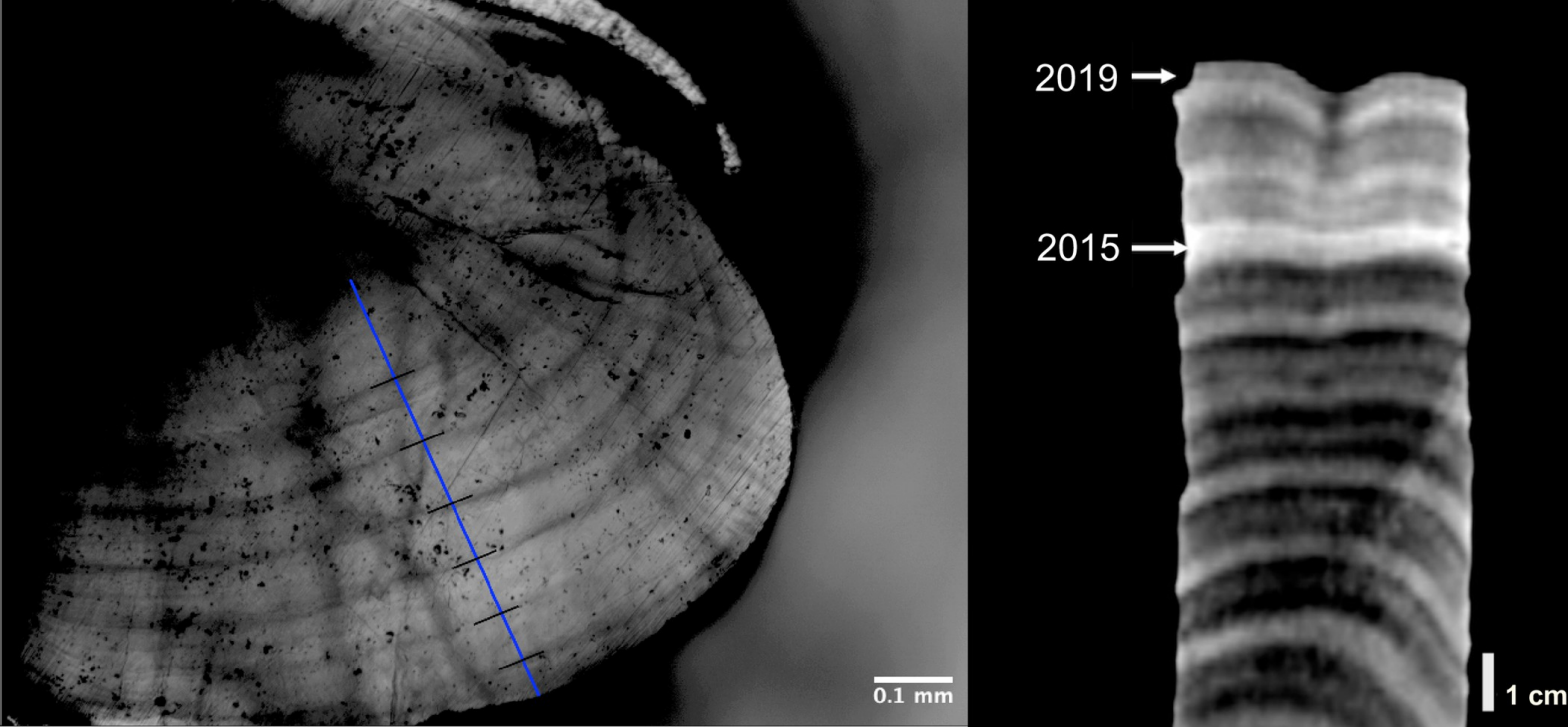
Examples of annual growth increments in an otolith (left) and coral core (right). Left: the otolith is a polished sagittal cross-section from *Pomacentrus sulfureus*. The blue line is drawn perpendicular to the growth lines, and parallel with the axis of growth. The black lines mark the annual increments. Right: a computed tomography (CT) scan of a *Porites* spp. coral from the Farasan Banks with one high density stress band (2015), among the annual density bands ranging from approximately 2009 until date of collection in 2019.
